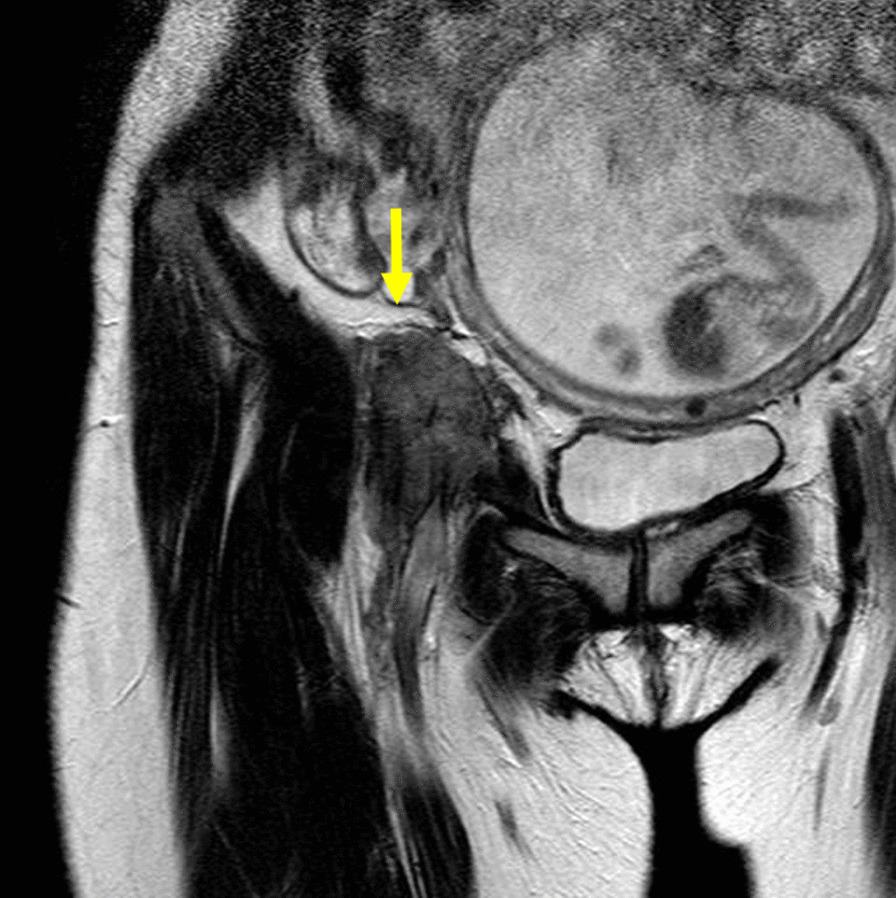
Coronal T2-weighted magnetic resonance imaging of the pelvis. The lesion in the right inguinal region shows iso- to slightly high intensity (yellow arrow)
